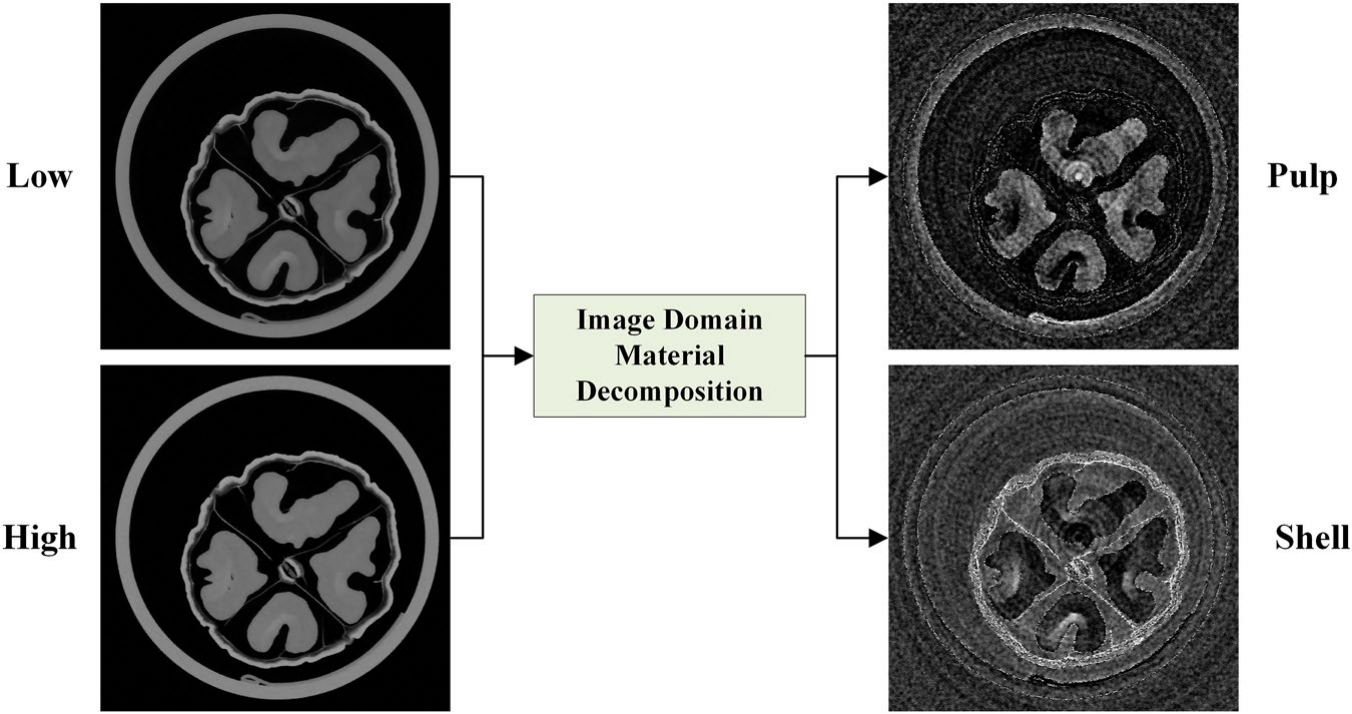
Material decomposition of walnut shell and pulp in the image domain. CT images display window: [−1000, 1000] HU, material decomposition maps display window:[−1000, 5000] mg/cm^3^.
